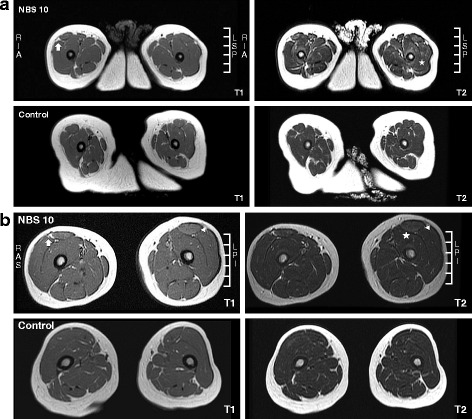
Muscle MRI in neonatally treated infants and toddlers with IOPD. a T1WIs (left panel) and T2WIs (right panel) of thigh muscle MRI scans obtained at 1 year of age from NBS10 (upper panel) and an age- and sex-matched control (lower panel) were compared. NBS10 presented hyperintensity at the bilateral quadriceps, particularly the vastus intermedius, on T2WIs (asterisk) but not on T1WIs. In addition, increased fat in the intramuscular fascia (between the vastus lateralis and rectus femoris) was also observed (arrow). b T1WIs (left panel) and T2WIs (right panel) of thigh muscle MRI scans obtained at 3 years of age from NBS10 (upper panel) and an age- and sex-matched control (middle panel) were compared. NBS10 presented hyperintensity at the bilateral vastus intermedius and less hyperintensity at the vastus lateralis on T2WIs (asterisk) but not on T1WIs, showing a significant decrease compared with previous images obtained at 1 year of age. In addition, hyperintensity at the bilateral rectus femoris (triangles) was clearly evident on both T1WIs and T2WIs. Increased fat in the intramuscular fascia (between the vastus lateralis and rectus femoris) was also observed but had not progress (arrow)
